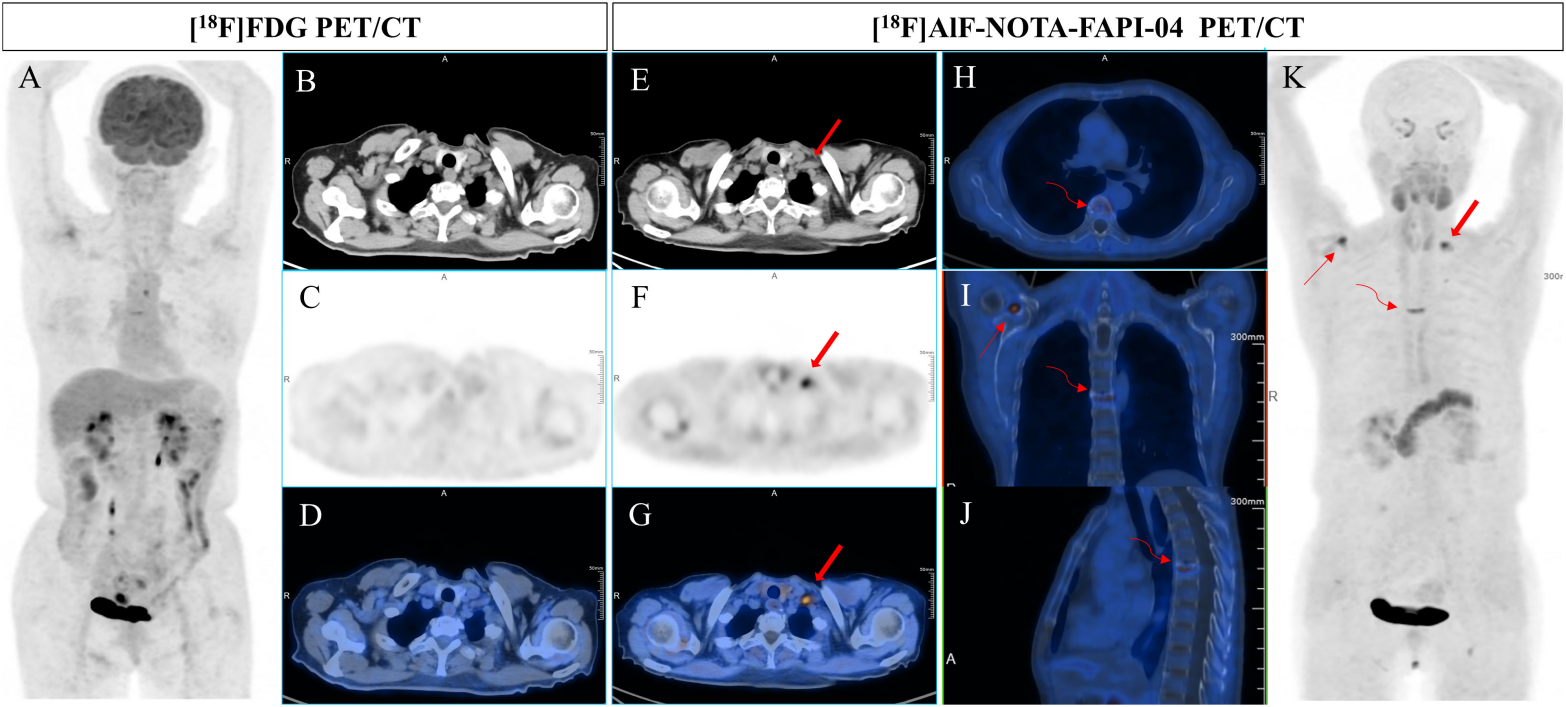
A 61-year-old woman underwent gastric radical resection for a cancer of nine months old and received 6 cycles of SOX therapy. Elevated levels of CEA, CA199, and CA50 (9.5 ng/mL, 98.35 U/mL, and 48.4 IU/mL, respectively) were detected during routine follow-up. To detect potential metastases, she underwent [^18^F]FDG PET/CT (A-D) examination, which revealed no abnormal uptake in the entire body. However, [^18^F]AlF-NOTA-FAPI-04 PET/CT (E-H) showed increased tracer uptake in the left supraclavicular lymph node (bold arrow, the SUVmax of 7.9). Additionally, subsequent contrast-enhanced CT demonstrated significant enlargement of the lesion. In addition, [18F]AlF-NOTA-FAPI-04 imaging revealed abnormal uptake in the degenerative bone disease of the sixth thoracic vertebra (H-K, curved arrow) and in the right shoulder joint due to arthritis (I, K, thin arrow), with SUVmax of 6.5 and 7.2, respectively.
